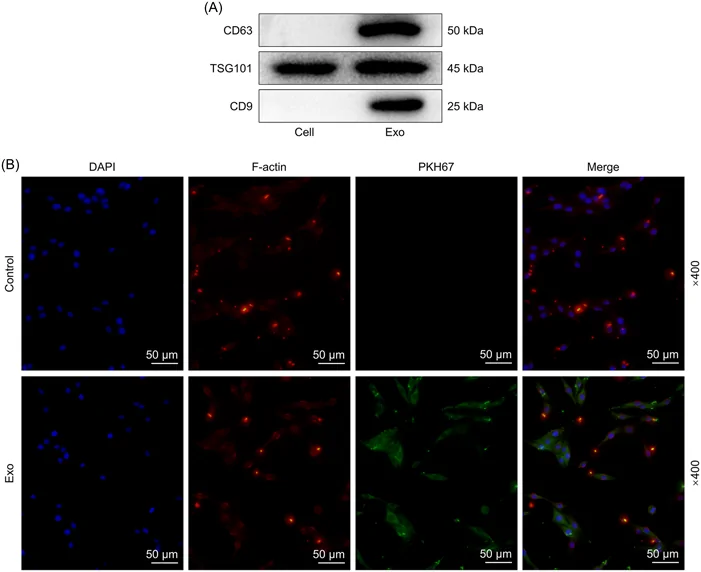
Exosomes isolated from HCC cells could be internalized into macrophages. (A) Exosomes were isolated from MHCC97‐H cells and identified by Western blot analysis, with antibodies against CD63, TSG101, and CD9. (B) The internalization of MHCC97‐H cell‐isolated exosomes into THP‐1 cells was observed after PKH67 labeling of exosomes (magnification: ×400; scale: 50 µm). DAPI, 4′,6‐diamidino‐2‐phenylindole; HCC, hepatocellular carcinoma; PSMA5, proteasome subunit alpha 5; shNC, short hairpin RNA against negative control; shPSMA5, short hairpin RNA against PSMA5; TSG101, tumor susceptibility gene 101.
